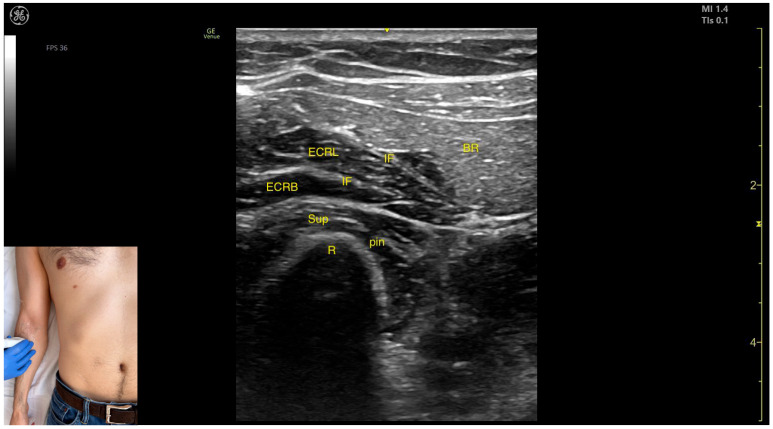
Ultrasound anatomy of the brachioradialis (BR) in the forearm compartment with key landmarks: BR—brachioradialis; IF—intermuscular fascia; ECRL—extensor carpi radialis longus; ECRB—extensor carpi radialis brevis; Sup—supinator; R—radius; and pin—posterior interosseous nerve.
